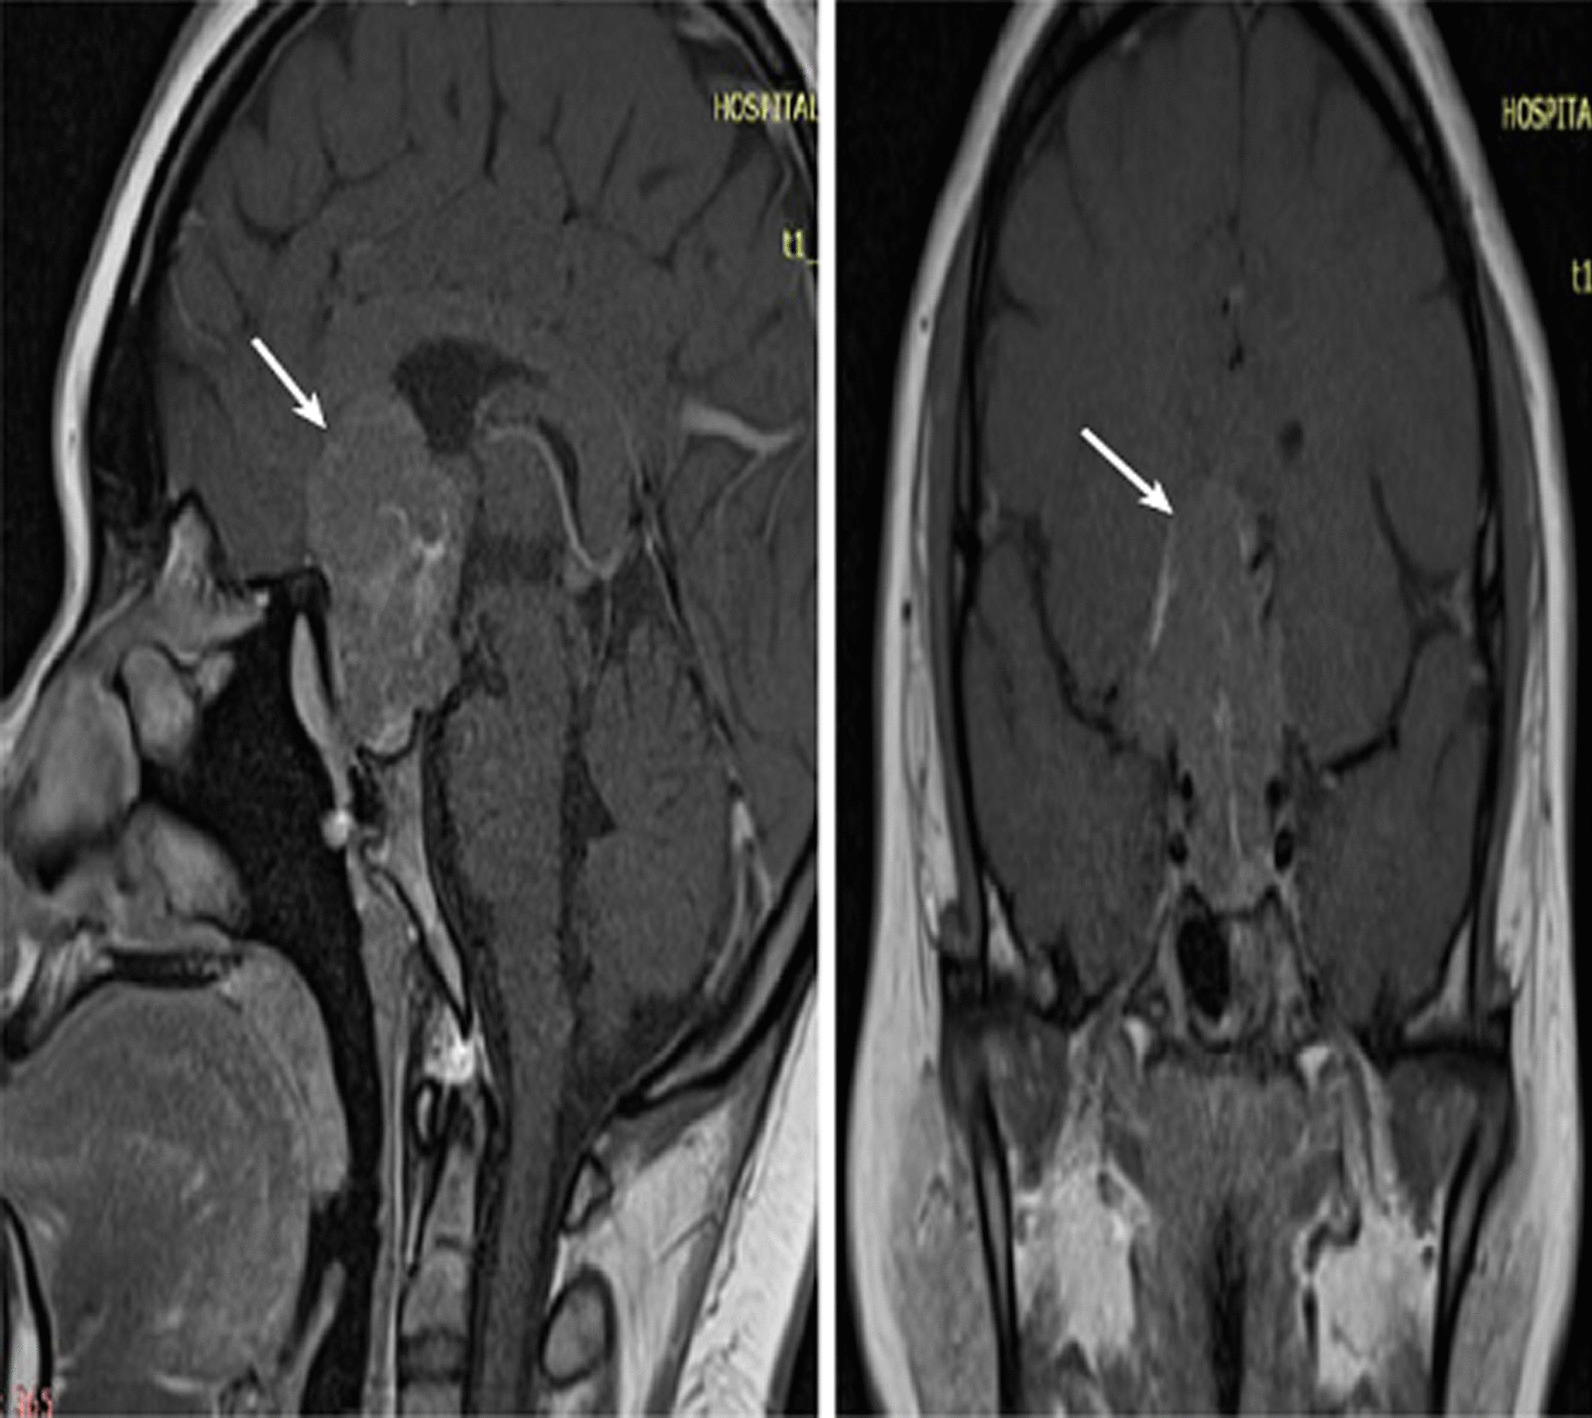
Preoperative sagittal and coronal T1-weighted magnetic resonance imaging pituitary performed prior to the third debulking surgery. MRI revealed an increased tumor size (2.6 × 3.0 × 3.7 cm^3^) with a larger suprasellar component (white arrow) compressing towards the corpus callosum, lateral ventricle, and third ventricle
